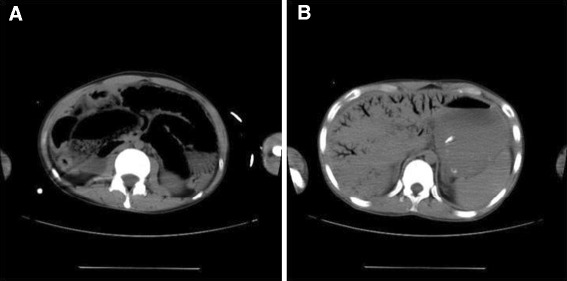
Computed tomography (CT) findings on the day of admission. The unenhanced CT scan shows diffuse gaseous distention with pneumatosis of the small bowel (A) and extensive hepatic portal venous gas (B).
